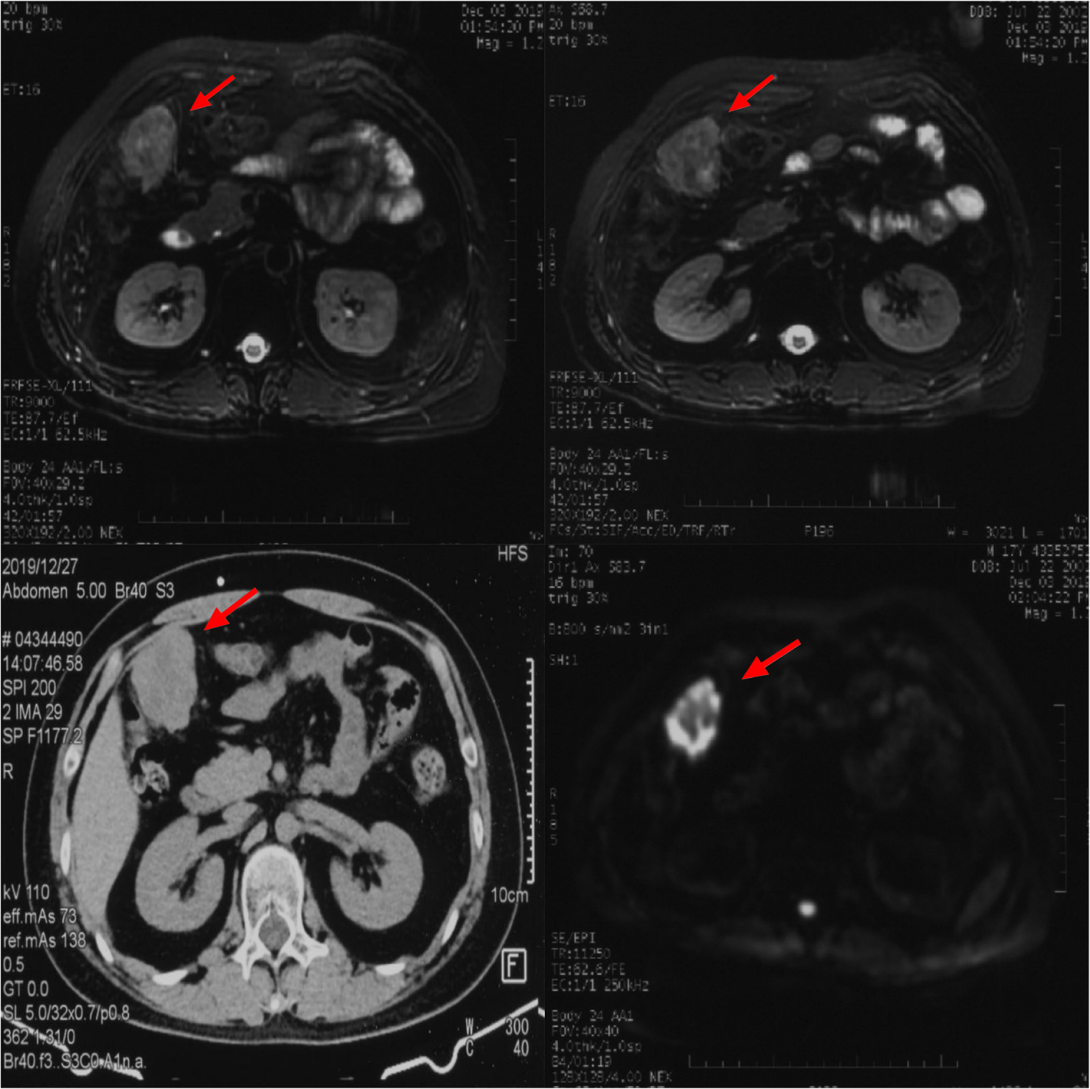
Contrast-enhanced MRI revealed rough-edged masses in the right upper abdominal mesentery with hyperecho and obvious heterogeneous enhancement. The red arrow shows the abnormal enhancement in this patient’s abdominal MRI
